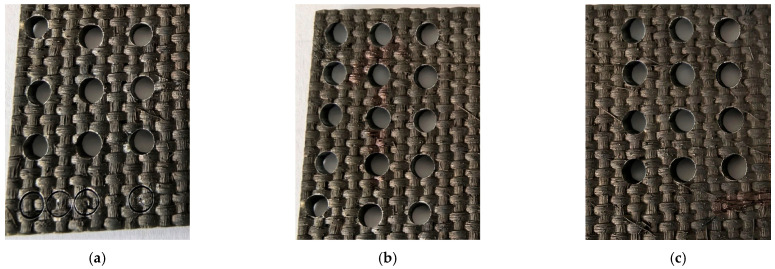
Drilled Sample. (a) Water-jet-guided laser; (b) ultrasonic grinding; (c) water-jet-guided laser-ultrasonic grinding.
